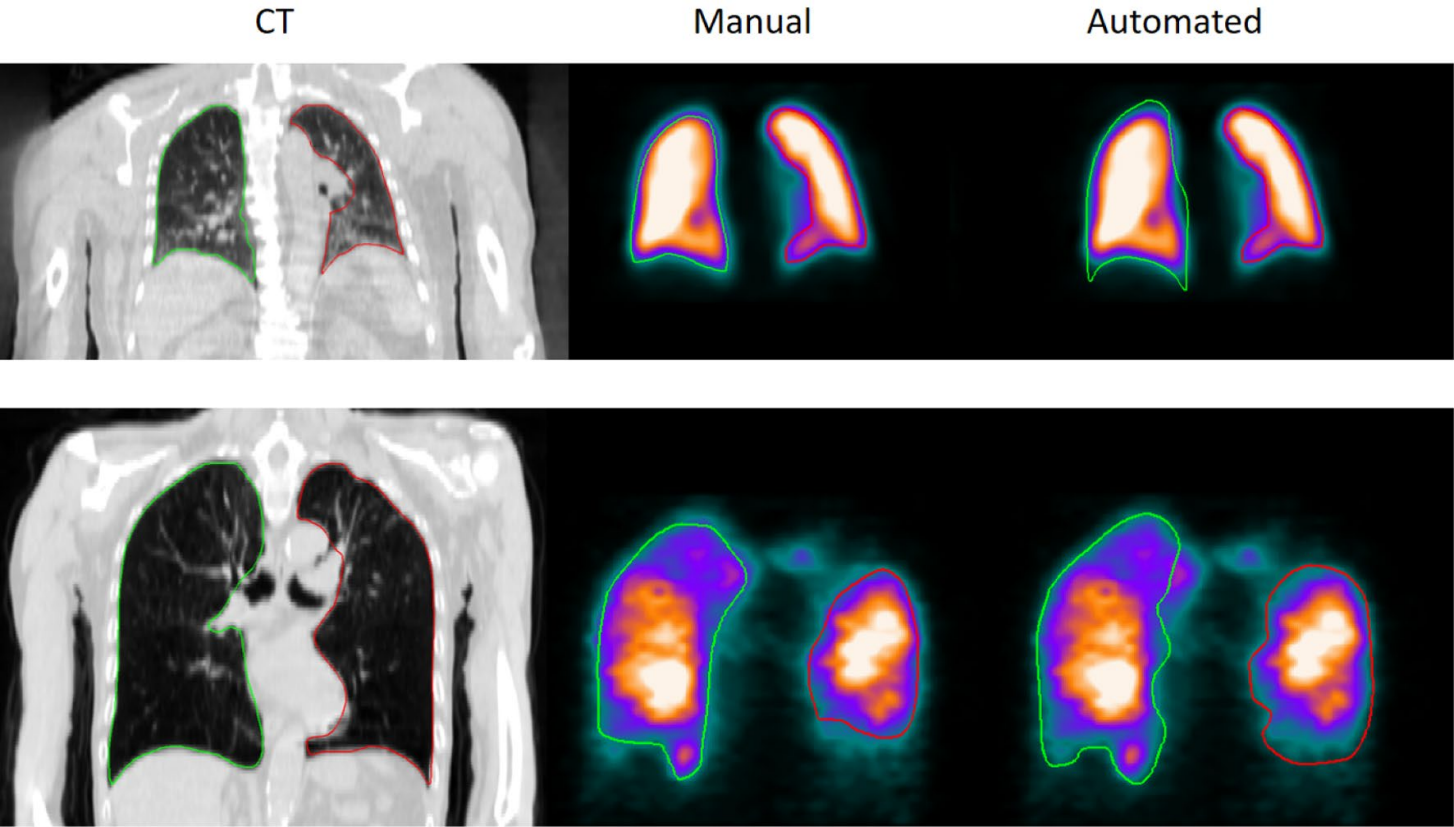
CT image, manual and automatic segmentation of two patients. Top row shows a slice from a patient with normal ventilation/perfusion and the bottom row shows a slice from a patient with peripheral loss of both ventilation and perfusion. The left column shows CT images, the middle column shows manual segmentations, and the right column shows automatic segmentations. Green delineation colour = left lung, red delineation colour = right lung
